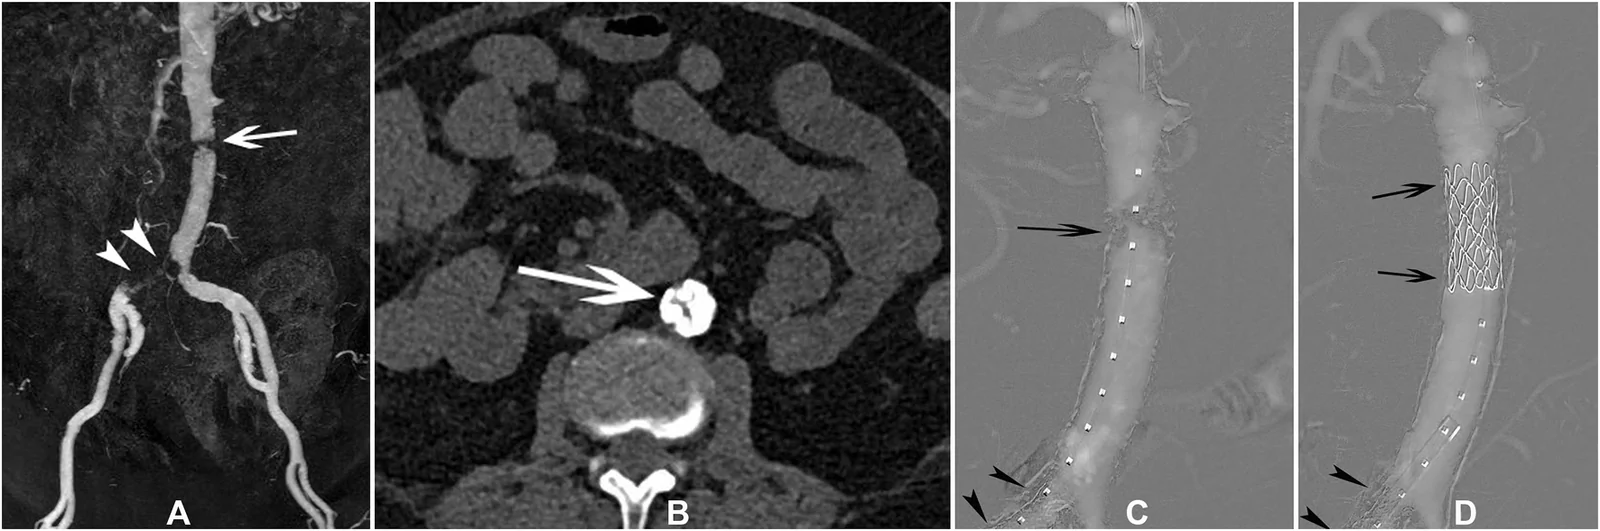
Hypertensive patient with serum creatinine decline (2.95 mg/dL) and focal stenosis in the distal aorta. (A) Magnetic resonance (MR) angiography demonstrates a focal, severe stenosis (arrow) in the infrarenal aorta; loss of signal in the right common iliac artery (arrowheads) due to stent artefact. (B) Unenhanced computed tomography confirms a circumferential and heavily calcified aortic stenosis (arrow). (C) Carbon dioxide (CO2) aortography reveals a focal, infrarenal aortic stenosis (arrow); note also the stent (arrowheads) in the common right iliac artery. (D) Carbon dioxide (CO2) aortography after endovascular intervention shows a fully expanded aortic stent (arrows); note also a stent in the proximal right common iliac artery (arrowheads).
